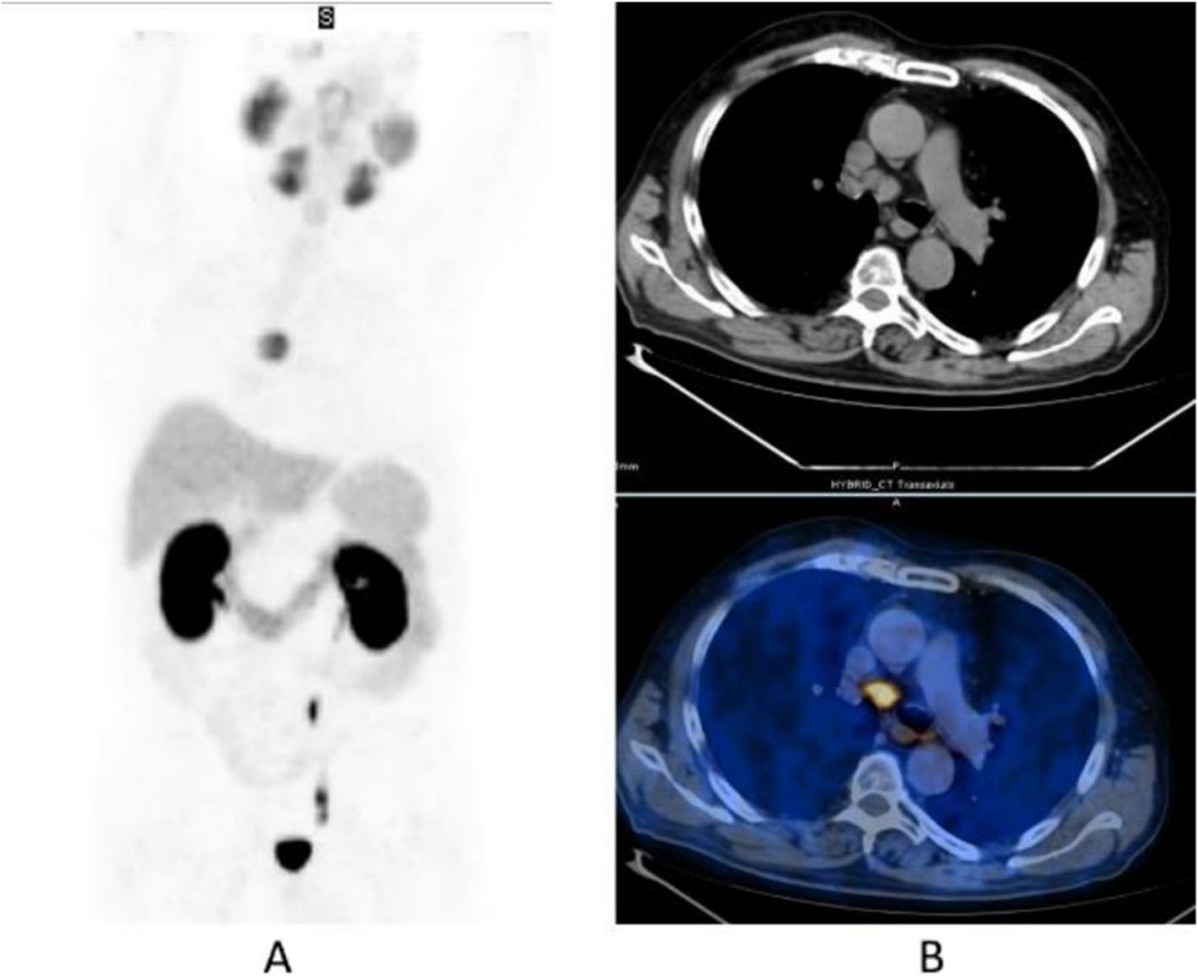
^68^Ga-PSMA, prostate cancer, biochemical recurrence (BCR): uncommon precarinal solitary metastasis *Clinical history*: 64 y.o. man with prostate cancer, radical prostatectomy as primary treatment Gleason score 4 + 5; PSA = 1.9 ng/ml, PSA dt 6 months, TTR 28 months. *PET/CT findings*: focus of increased uptake seen in an enlarged precarinal lymph node (24 mm). Transbronchial biopsy diagnosed prostate cancer relapse. a MIP images; b CT and fused images
